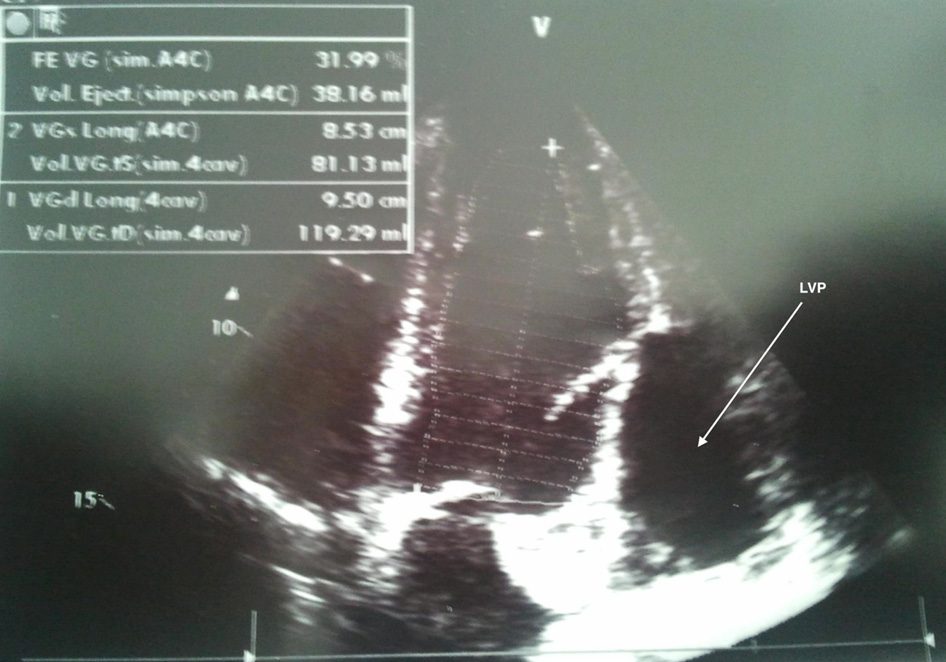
Echocardiography view showing the left ventricular pseudoaneurysm (LVP)
